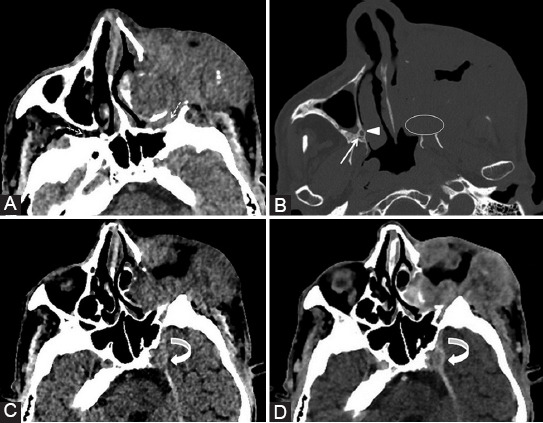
The left maxillary squamous cell carcinoma with orbital extension. Axial computed tomography (CT) image (A) reveals the obliteration of PPF fat pad on the left compared to the right side (dashed arrows). Bone window axial CT scan (B) shows complete involvement of the left palatine foramina (ellipse) versus the right greater (arrowhead) and lesser (arrow) foramina. Axial CT before (C) and post-contrast (D) administration demonstrates left Meckel’s cave tissue effacement and contrast enhancement at this level (curved arrow).
